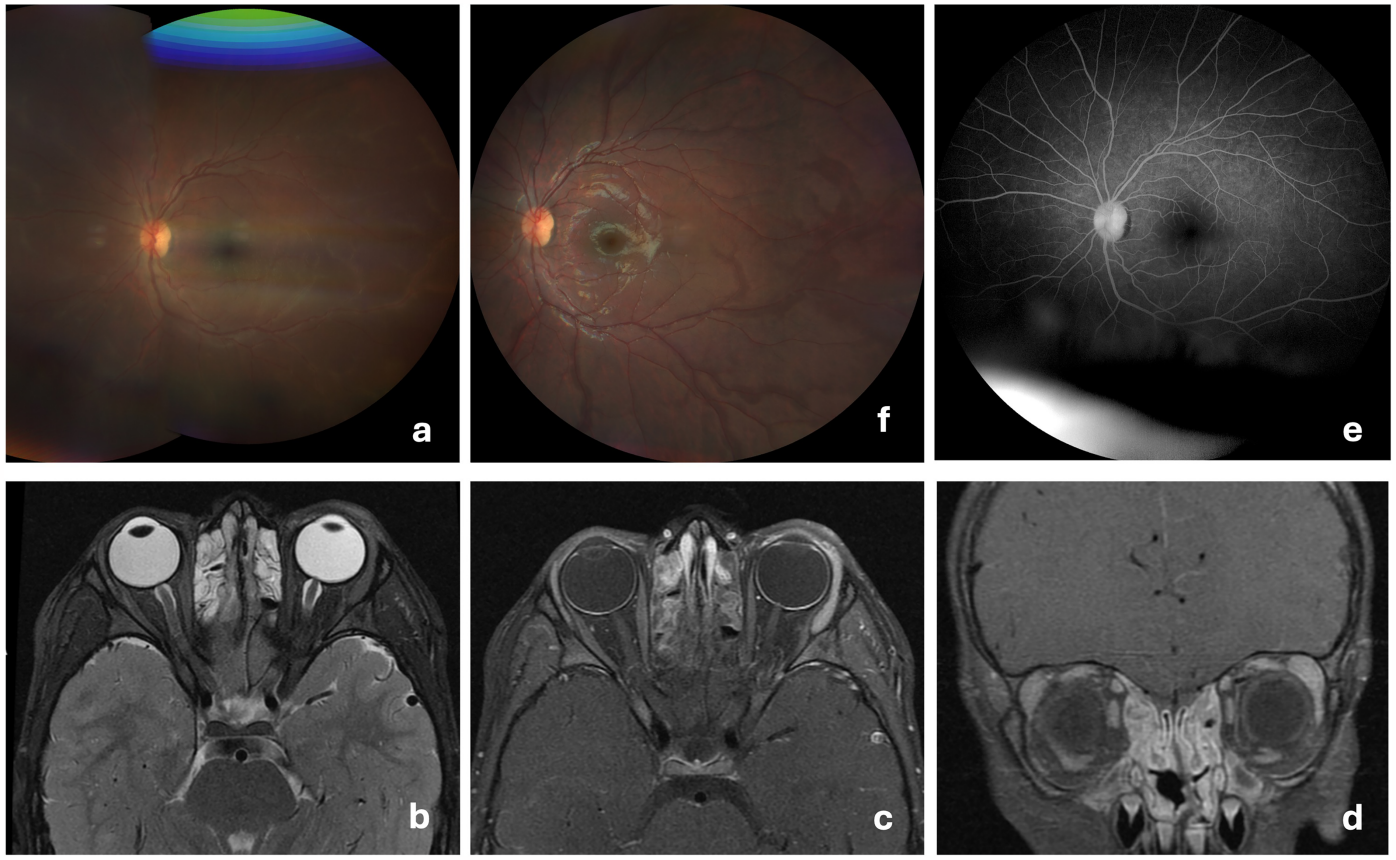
Retinal vasculitis and MRI findings at admission with follow-Up resolution. (a) At admission, fundus image of the left eye showing retinal arterial vasculitis, characterized by vessel wall irregularities, attenuation of the arteries, and signs of perivascular exudation; (b–d) Orbital MRI at admission shows enhanced contrast uptake in the left uveal tract (iris, ciliary body, and choroid) with mild anterior prominence at the optic disc. Anterior globe margin enhancement suggests conjunctival inflammation. Increased volume and contrast uptake in the left lacrimal gland indicate adenitis. Evidence of rhinosinusitis; (e) Two months post-discharge reassessment of the left eye, showing no evidence of sequelae on fundoscopic examination; (f) Two months post-discharge reassessment, with angiography documenting the resolution of vasculitis.
